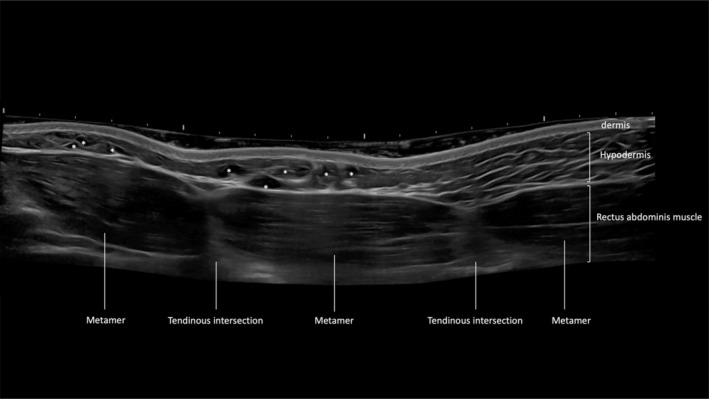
B‐mode ultrasound image of the abdomen (longitudinal view) showing the dermis, hyaluronic acid deposits (*) volumizing the hypodermis, the rectus abdominis muscle divided by the tendinous intersections in metameres.
